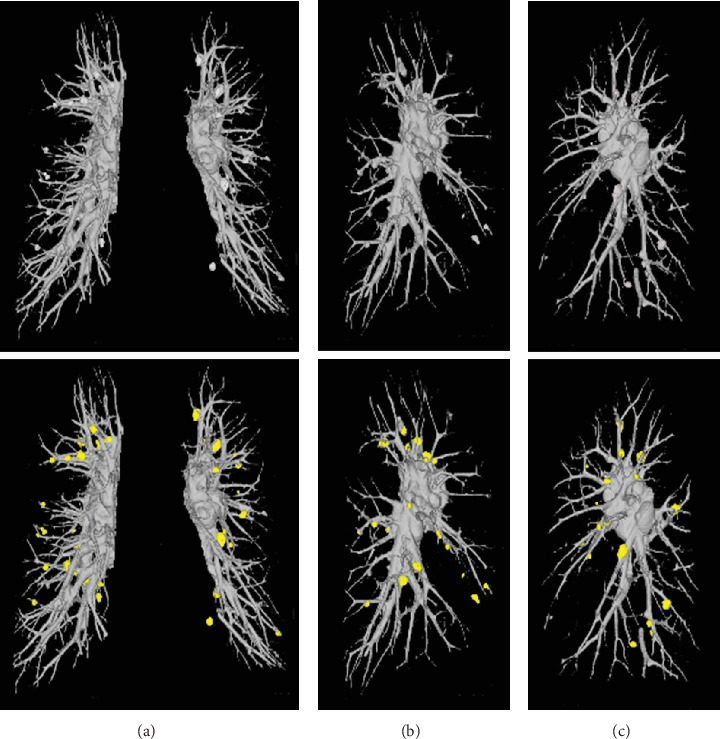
On 3D images created from the CT, pulmonary nodules and pulmonary vessels extracted from the original CT are shown in light gray (upper panels of (a), (b), and (c)). In the fusion images (lower panels), where the original CT and the vessel-suppressed CT are superimposed, the nodules extracted in the vessel-suppression image are shown in yellow. These reconstructions demonstrate the spatial relationship between pulmonary vessels and multiple nodules, providing three-dimensional visualization of nodule distribution. (a) Frontal view of both lungs showing original and fusion images. (b) Lateral view (RAO at 90°) of the right lung displaying original and fusion images. (c) Lateral view (LAO at 90°) of the left lung showing original and fusion images.
